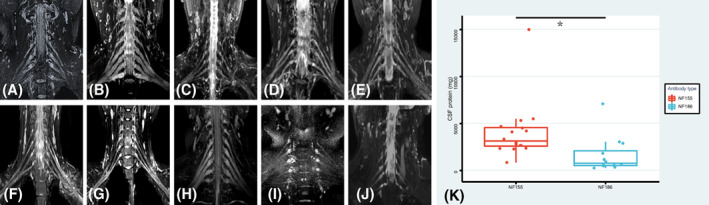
Brachial plexus MRI of anti‐NF186+ patients and CSF protein level of anti‐NF186+ and anti‐NF155+ patients. (A) Brachial plexus MRI of healthy control; (B) Typical brachial plexus MRI of anti‐NF155+ neuropathy; (C–J) Brachial plexus MRI of P1, P2, P3, P4, P7, P8, P12 and P13 with anti‐NF186 antibody. (K) Comparation of CSF protein level between anti‐NF186+ and anti‐NF155+ patients (p* < 0.05).
